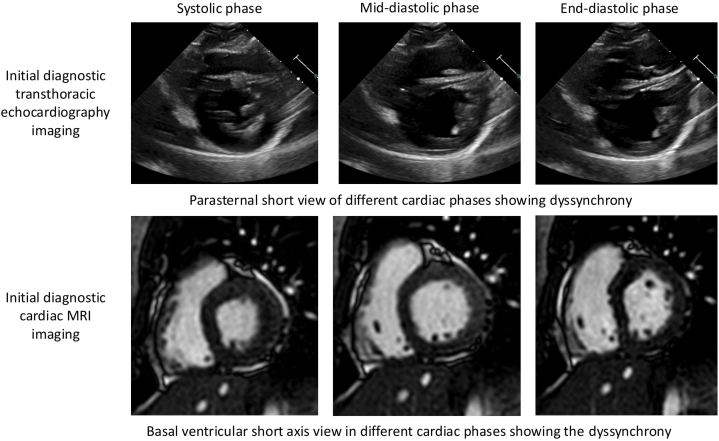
Still Transthoracic Echocardiogram and Cardiac MRI Images Demonstrating Dys-synchrony MRI = magnetic resonance imaging.
